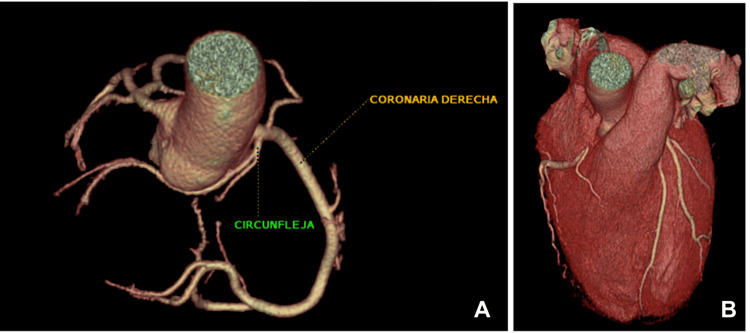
Computed tomography angiography of the heart coronaries (A) and myocardium (B) Coronaria derecha: right coronary artery; circunfleja: circumflex Original image by the authors
